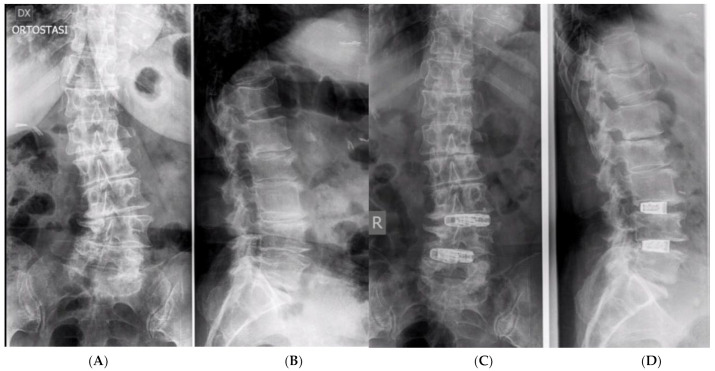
A 74-year-old woman. (A,B). Multiple DDD with left convex scoliosis and segmental L3-L5 kyphosis. (C,D) Postoperative X-rays showing two stand-alone expandable OLIF cages at L3-L4 and L4-L5.
